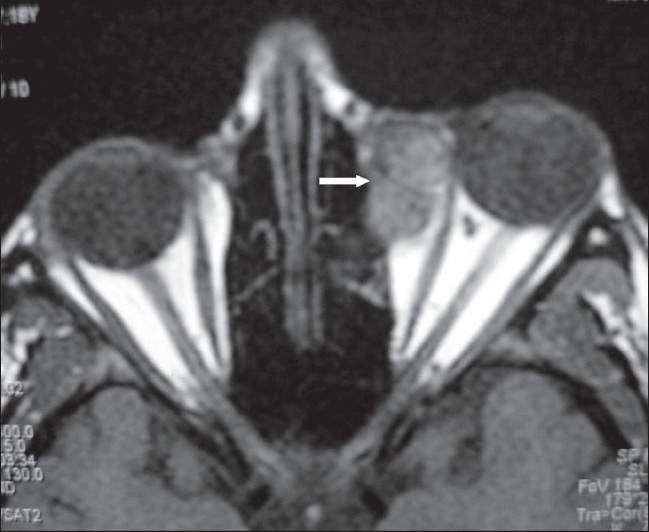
Axial T1W MRI image shows a well-defined extraconal lesion (arrow), isointense to the extraocular muscles, displacing the medial rectus laterally, anteriorly
